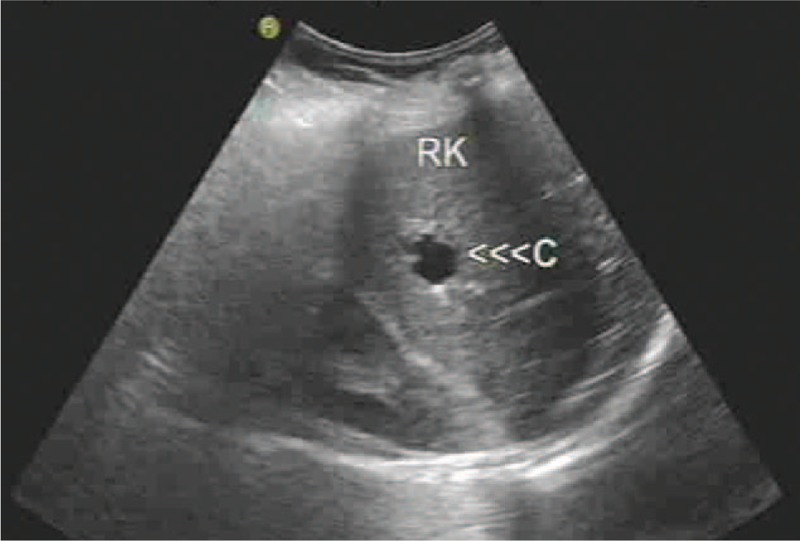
Abdominal sonogram revealing a liver cystic lesion with thin wall and smooth margin in the right liver lobe.
